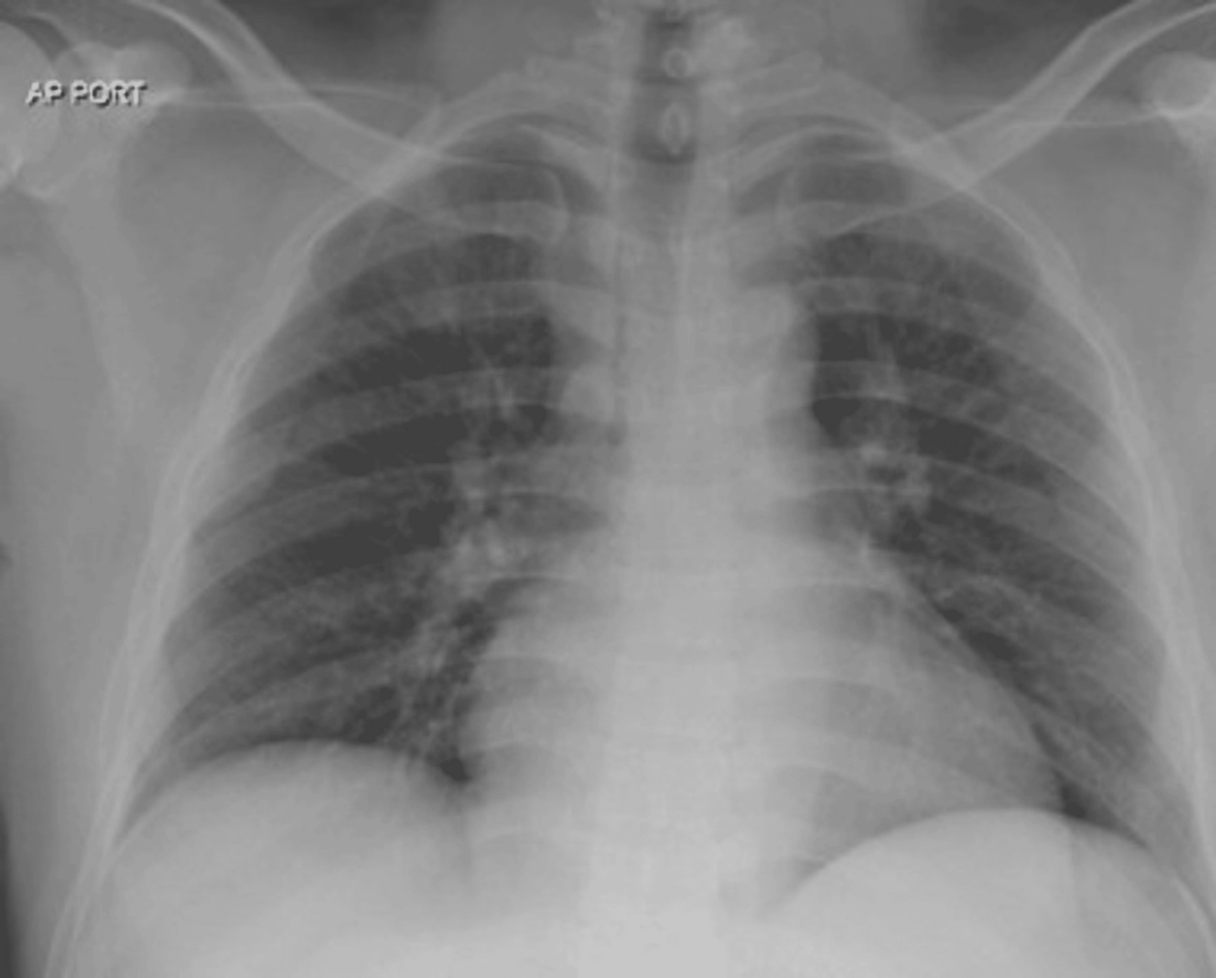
Chest X-ray, AP view, revealed an unremarkable study.
